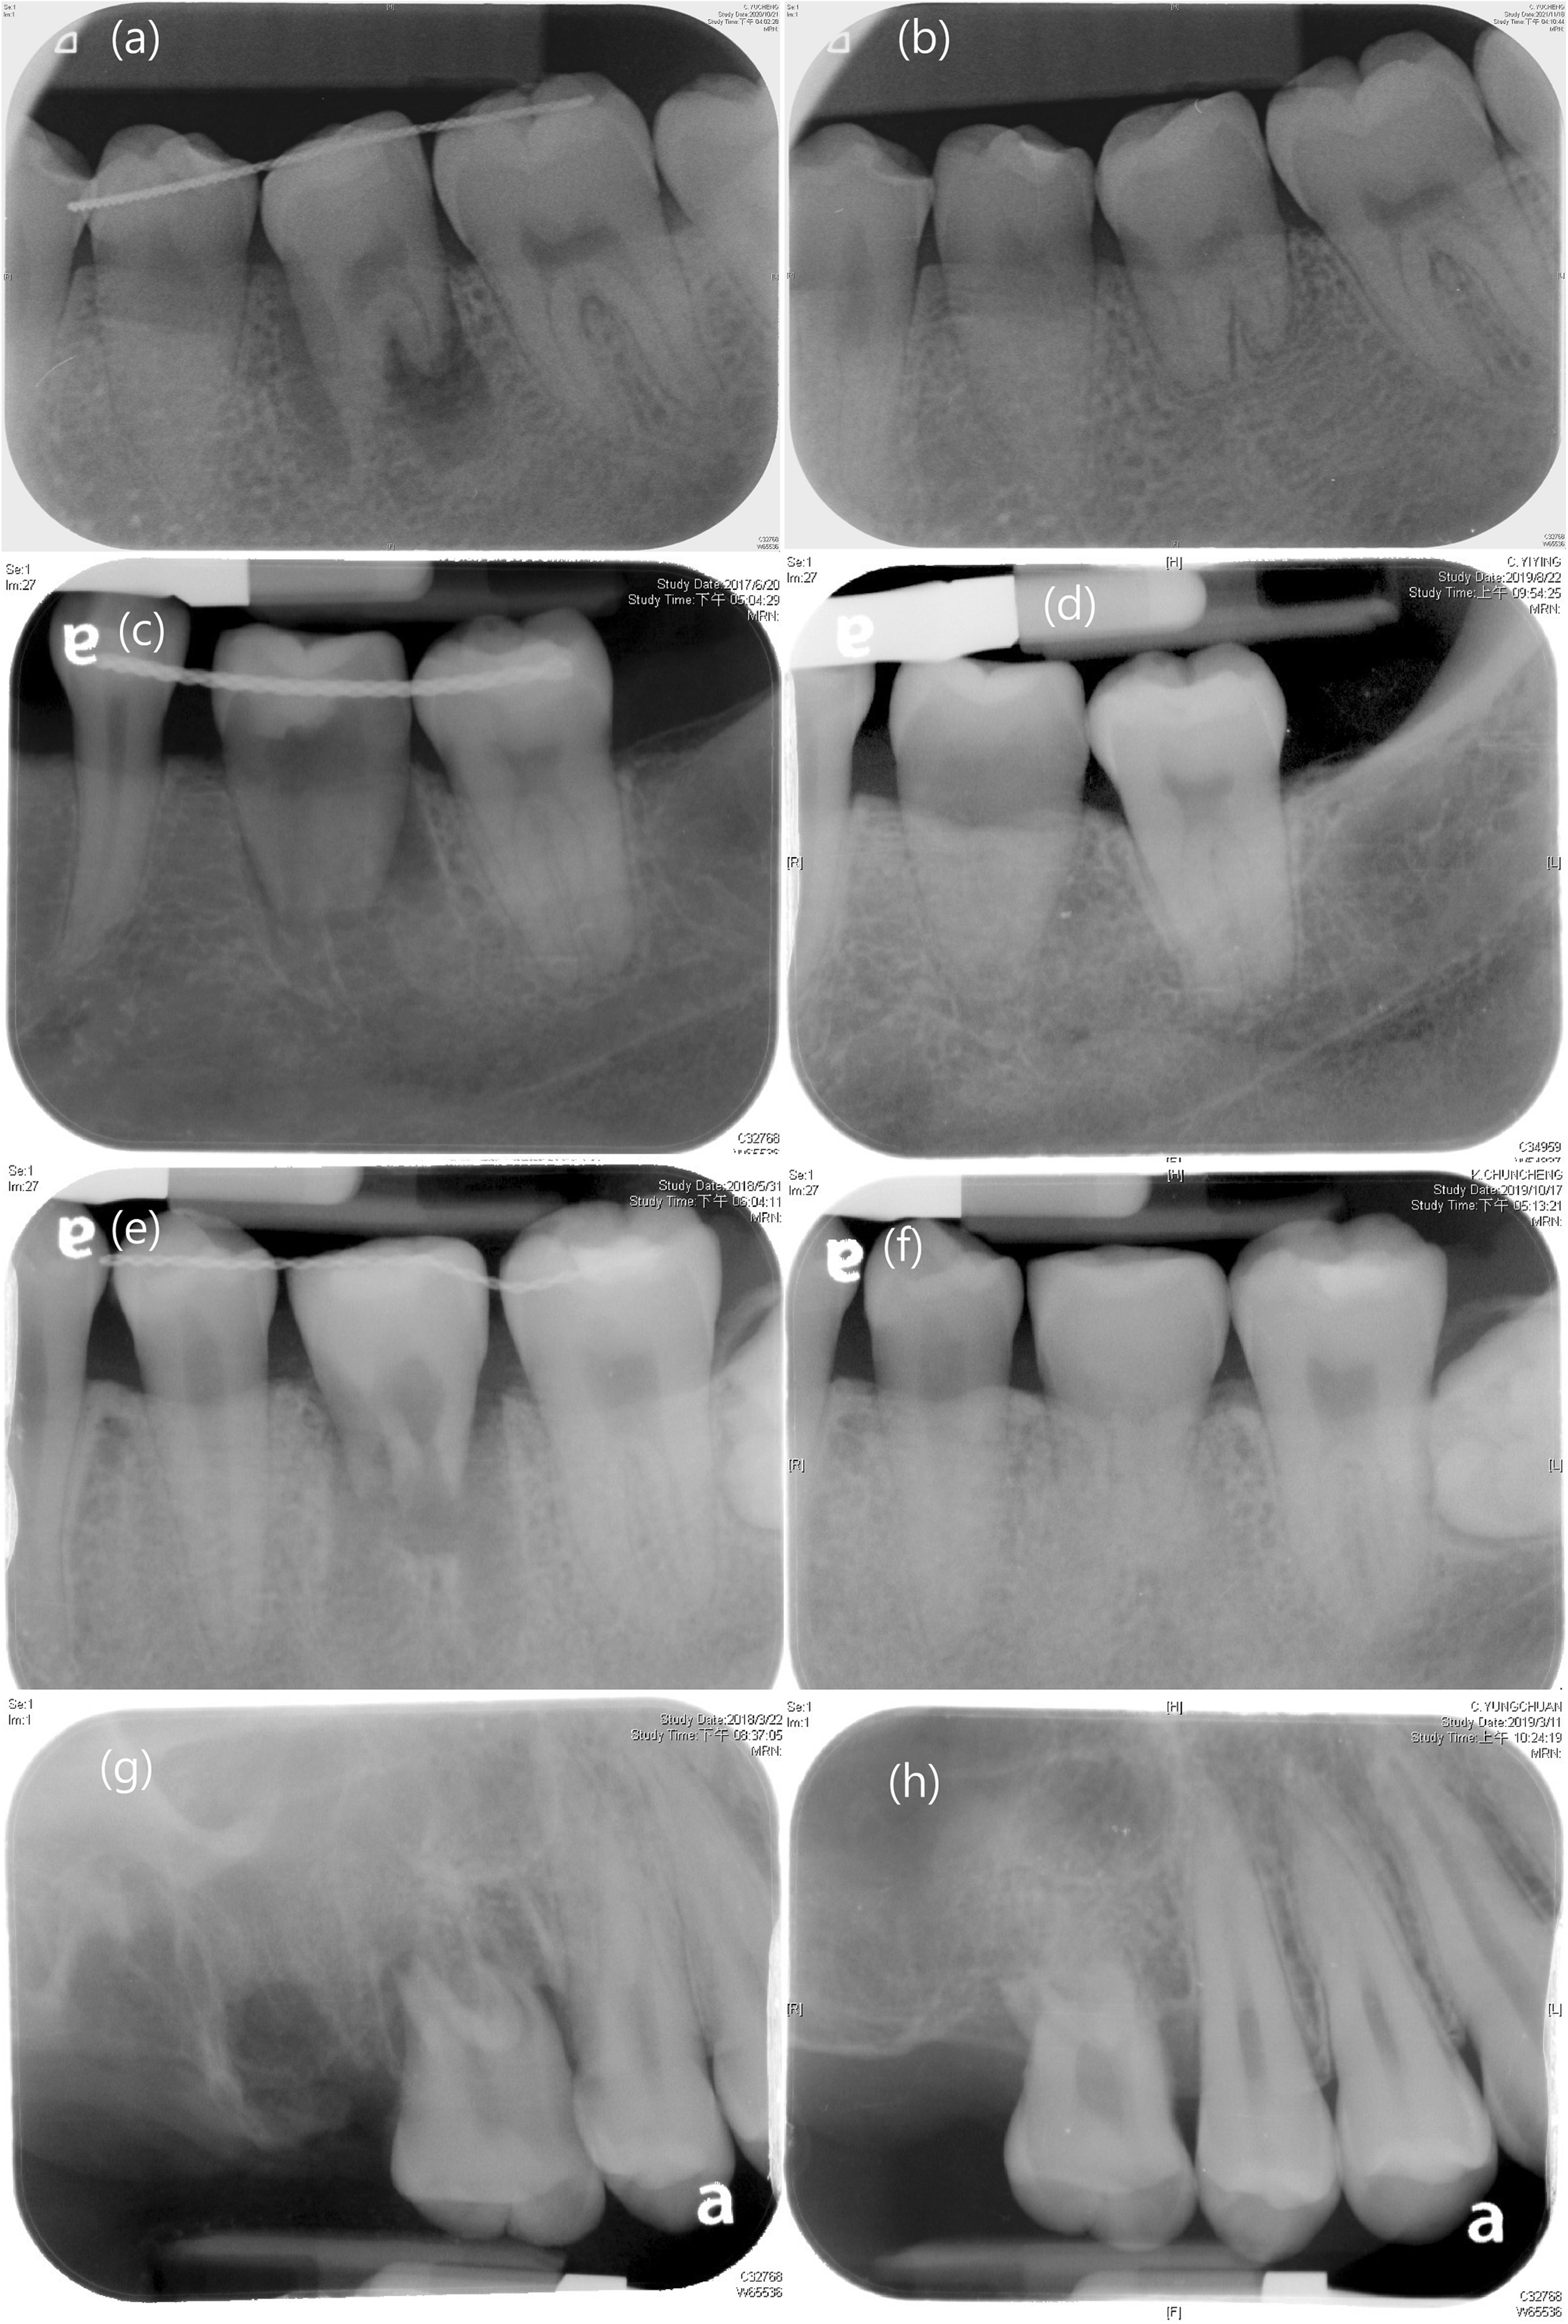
X-ray image of the transplanted tooth. a Patient 1, operation day. b Patient 1, 1 year after the operation. c Patient 2, operation day. d Patient 2, 2 years after the operation. e Patient 3, operation day. f Patient 3, 1.5 years after the operation. g Patient 5, operation day. h Patient 5, 1 year after the operation
